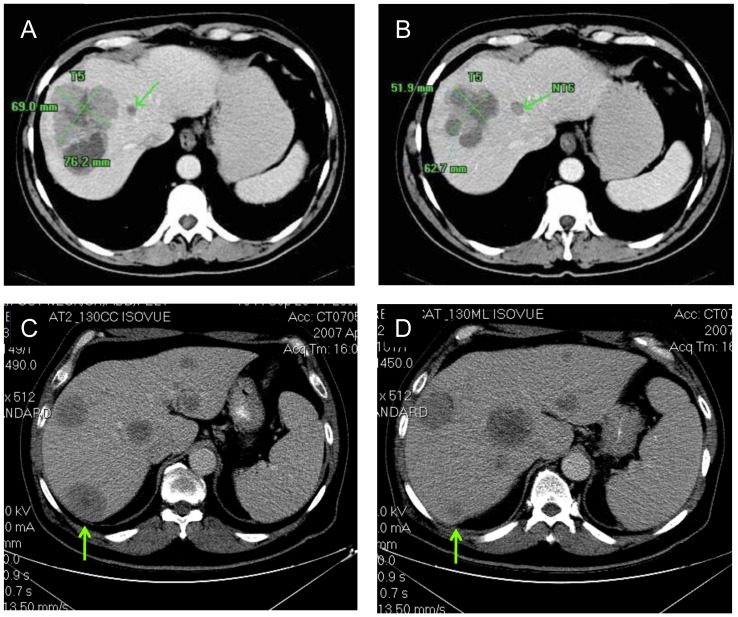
Mixed-tumor responses in patients with metastatic melanoma receiving GC1008. Patient 003 was previously treated with dacarbazine and sorafenib and then received GC1008 0.1/kg ×4 doses followed by 1 mg/kg ×4 doses on Extended Treatment. Representative CT scans comparing baseline (Panel A) and day 112 (2 months post Extended Treatment, Panel B) demonstrate a reduction in size of a liver metastasis (arrows) with features consistent with increased necrosis and cyst formation; however, a pulmonary hilar lesion demonstrated growth (not shown). Patient 008 was previously treated with multiple surgeries, high dose interleukin-2, and an investigational anti-melanoma vaccine and then received GC1008 1 mg/kg ×4 doses followed by 3 mg/kg ×4 doses on Extended Treatment. Comparison of CT scans from baseline (Panel C) and day 91 (Panel D) demonstrate near resolution of a liver metastasis (arrows); however, growth was noted in other hepatic lesions.
